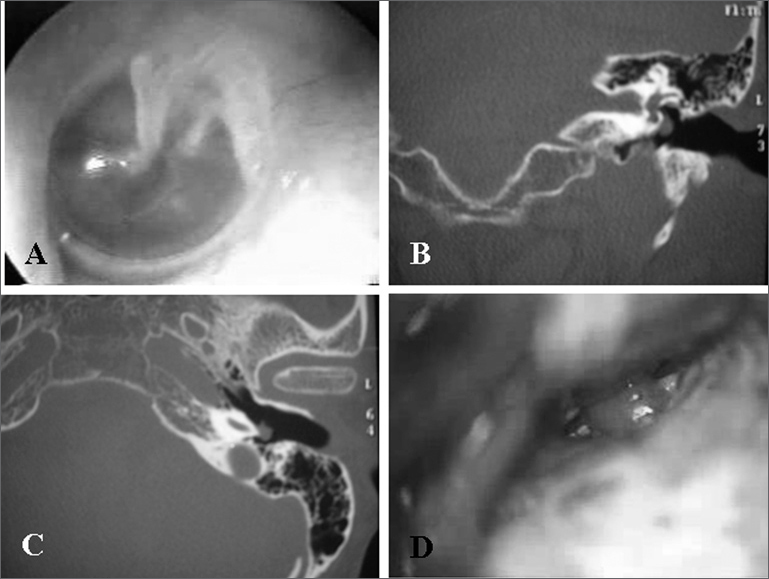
Tympanic paraganglioma (Case 1). Tumor touching the tympanic membrane (A); Temporal bone CT Scan, coronal (B) and axial (C) views, showing a soft tissue density mass on the left side, over the promontory and below the oval window; intraoperative photograph, retroauricular and transcanal approach showing the long process of the incus and the paraganglioma occupying part of the tympanic cavity(D).
